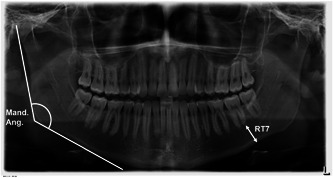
Orthopantomogram with acquired measurements. Distance of root tip of second molar (RT7) to mandibular base and jaw angle (Mand.Ang.).
